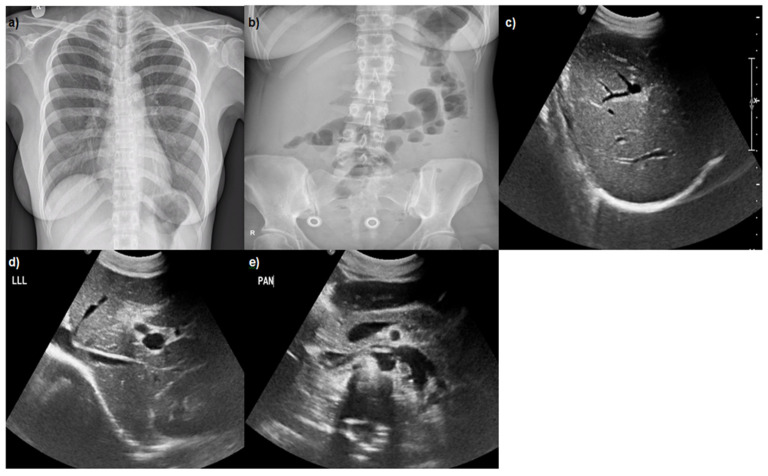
(a) Normal chest X-ray; (b) abdominal X-ray: mild air–fluid levels; (c–e) abdominal ultrasound: hepatomegaly, wall-thickening of gall bladder without stones, ‘starry sky’ appearance of parenchyma, and findings of hepatitis.
